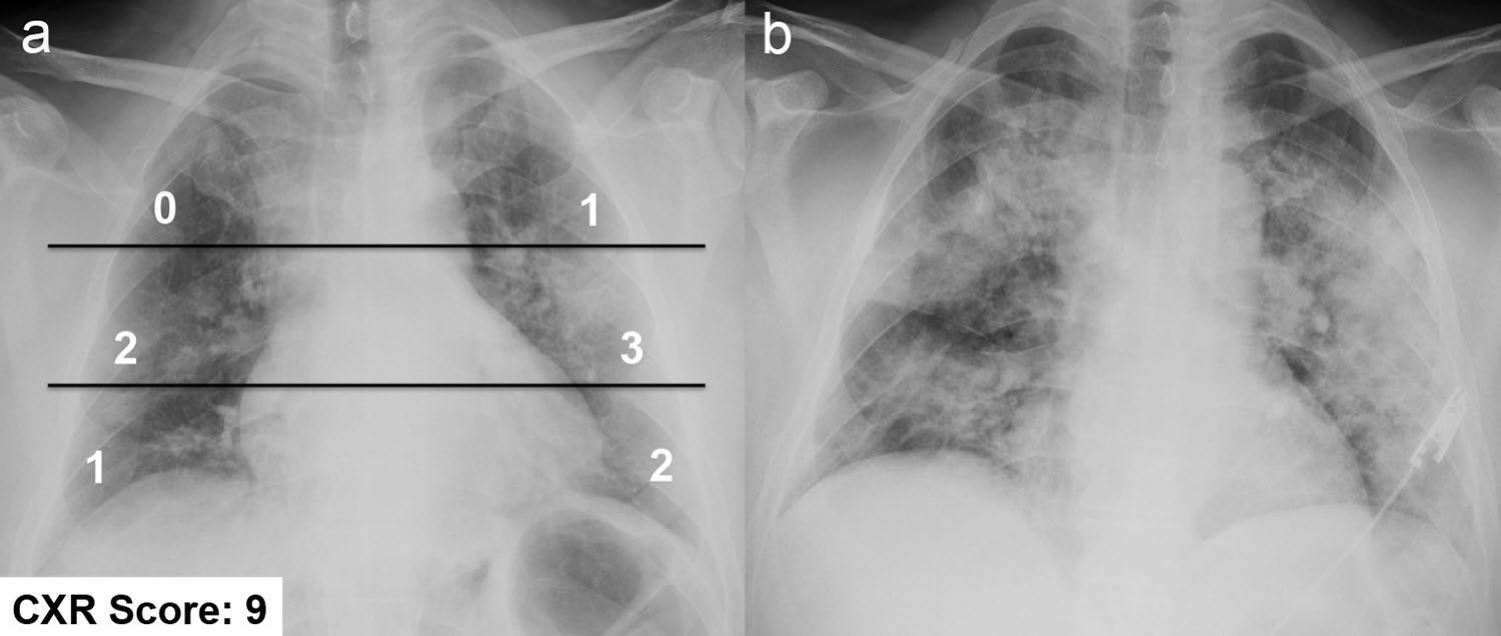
Bedside chest X-ray performed at the emergency department admission in a 62-years-old male affected by COVID-19 pneumonia (a), showing a Brixia score of 9 [67]. Note that the upper line is drawn at the level of the inferior wall of the aortic arch, while the lower line is drawn at the level of the inferior wall of the right inferior pulmonary vein. Based on the chest X-ray severity score (≥ 8) and age (≥ 50 years-old), this patient had an increased risk of developing a severe disease course. Indeed, the chest X-ray performed 6 days later (b) depicts a clear disease progression, with increase in density and extent of the abnormalities in both lungs. Lately, the patient recovered after ICU admission
